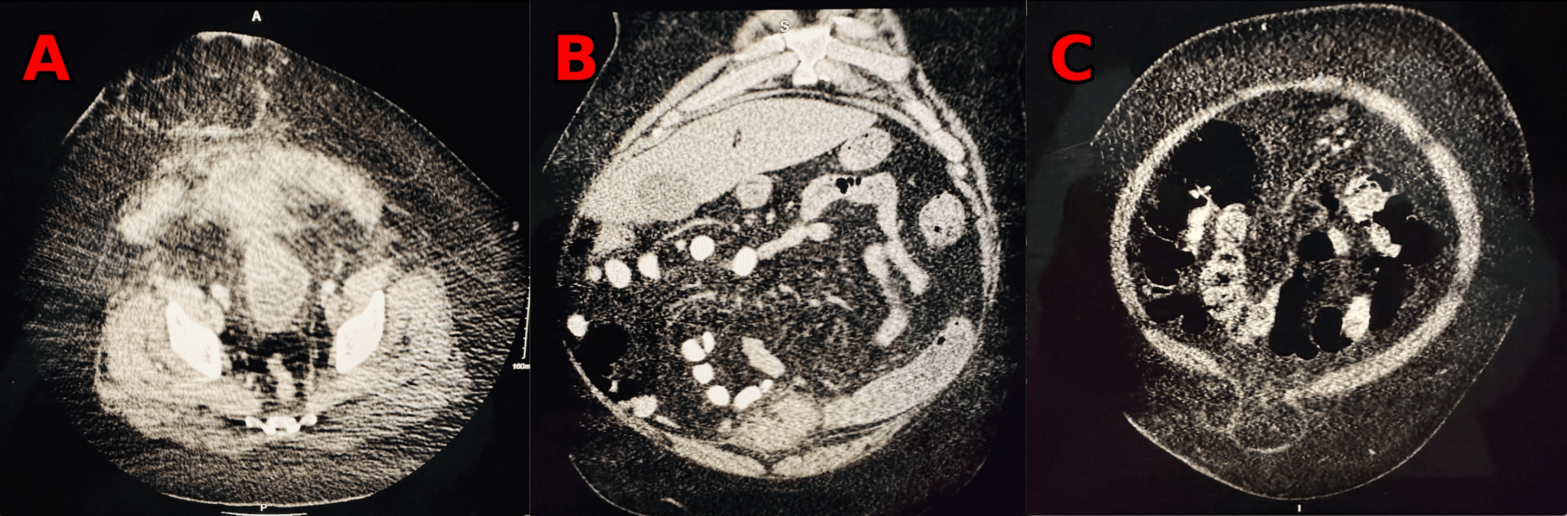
Initial CT scans demonstrating perforated diverticulitis and abdominal wall abscess with necrotizing fasciitis (A) Abdominal CT scan (axial) showing perforated diverticulitis with colocutaneous fistula and necrotizing fasciitis of the abdominal wall. (B) Abdominal CT scan (coronal) showing perforated diverticulitis. (C) Abdominal CT scan (coronal) showing perforated diverticulitis in a ventral hernia
